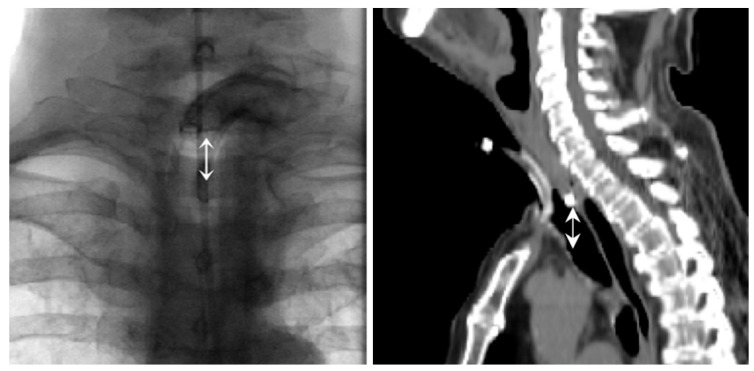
Barium swallow and CT scan (sagittal plane). Distance between lower voice prosthesis margin and manubrium ridge.
